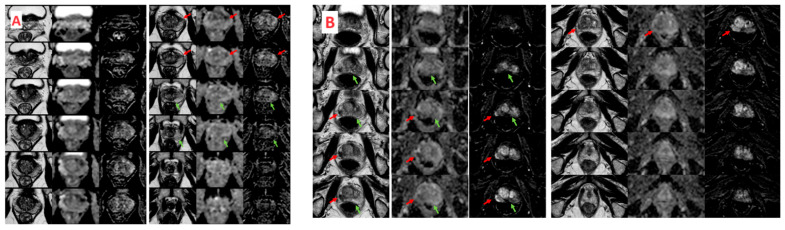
(A): *Out-field positivity in the same lobe of the MRI target.* MRI detected a PIRADS 4 lesion (red arrow) in the left anterior peripheral zone at the mid-portion of the prostate. This finding was confirmed by fusion biopsy, which identified ISUP 3 adenocarcinoma in the target area. Additionally, a focus of ISUP 3 adenocarcinoma was found in the left paramedian apical region where the MRI was negative (green arrow). (B): *Out-field positivity in the lobe contralateral to the MRI target.* MRI detected a PIRADS 5 lesion in the right peripheral zone in the posterolateral sector of the basal and middle zone (red arrow). Fusion biopsy identified ISUP 5 adenocarcinoma in the target area. Additionally, ISUP 5 adenocarcinoma was also found in the contralateral lobe, in the left peripheral zone at the base in the posteromedial sector, where the MRI (green arrow) showed no significant changes.
